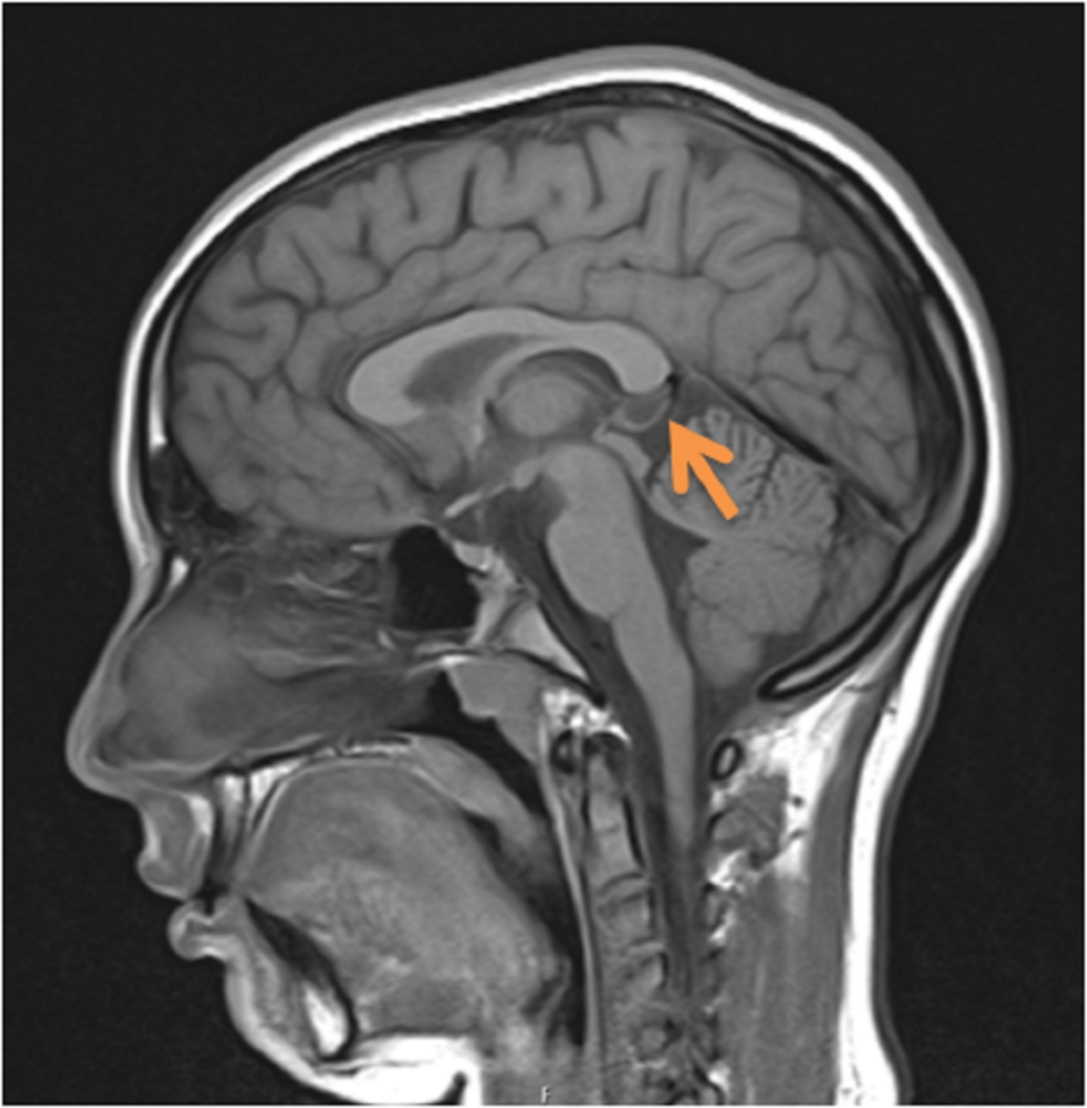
MRI brain sagittal section showing a small cyst of the pineal gland and no other relevant structural abnormalities.
